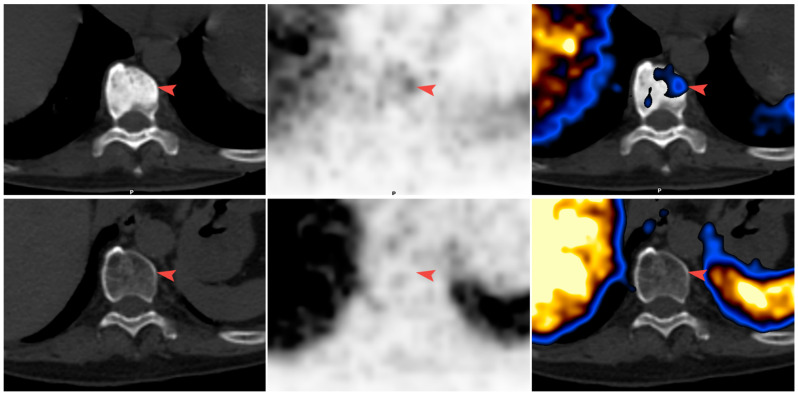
Disease response (Patient 7). (From left to right) CT; PET; and fusion images. In the first scan after ADT and bisphosphonate treatment, sclerotic metastasis in the vertebra was identified with very low uptake of 68Ga-PSMA-11 (arrowhead) (top row). In the second scan after ADT, the vertebra was no longer sclerotic and showed no uptake (bottom row). PSA levels were reduced and there was no evidence of new disease.
